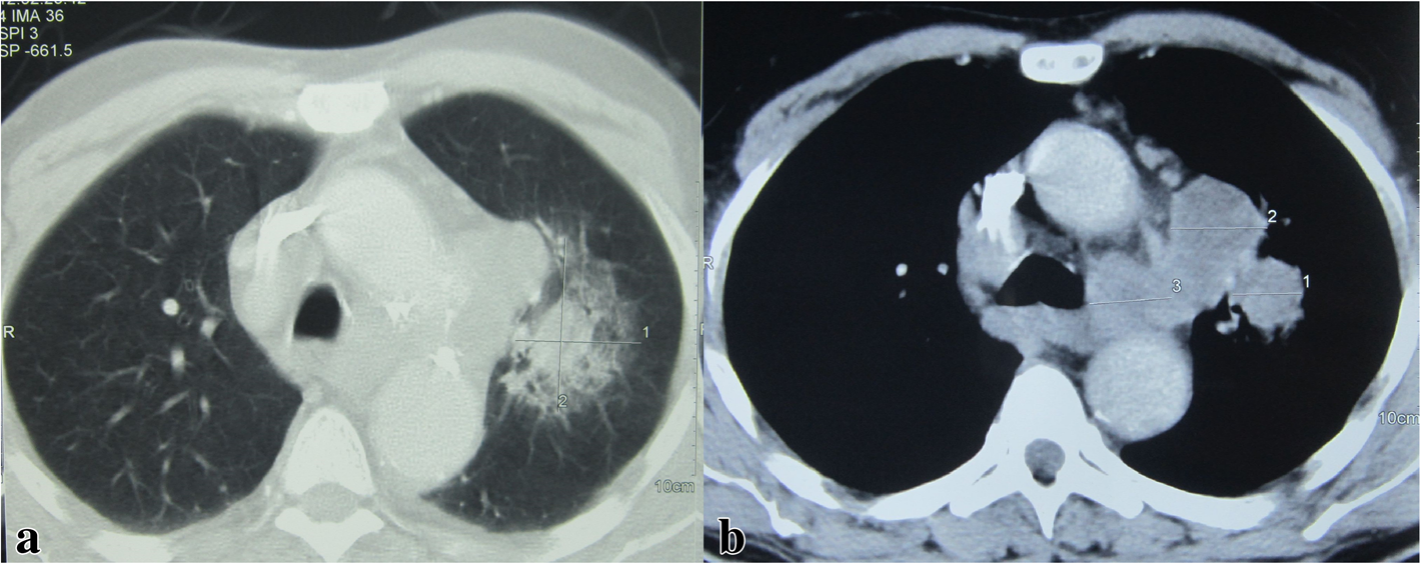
Initial chest CT. Attenuation in the left upper lobe and contralateral nodule on the parenchymal window (a). Bilateral adenopathy on the mediastinal window (b)
